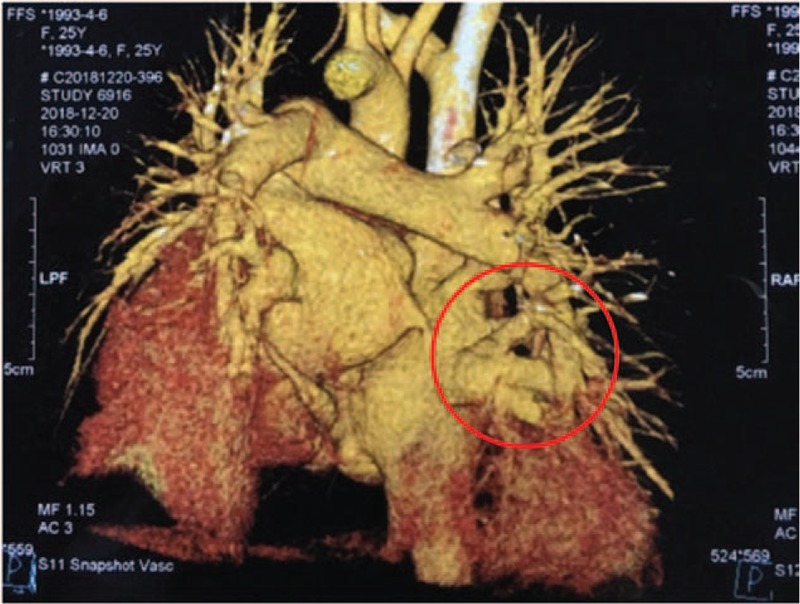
CTA shows right superior pulmonary vein, right middle vein, and right inferior pulmonary vein went into right atrium directly. CTA = computed tomography angiography.
